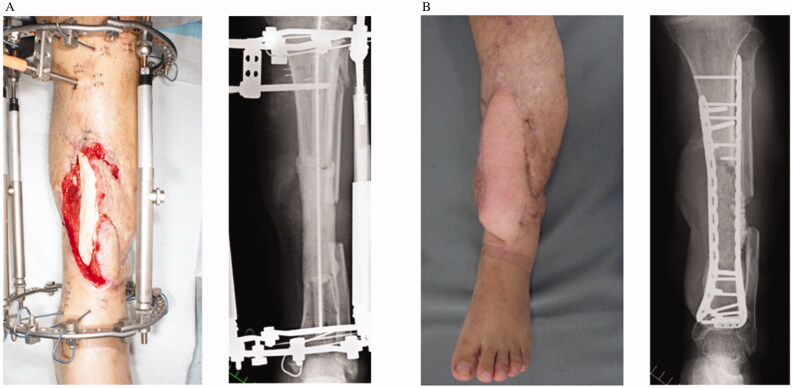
Case 1: (A) Preoperative clinical and radiographic findings. (B) Postoperative findings at 1 year.
